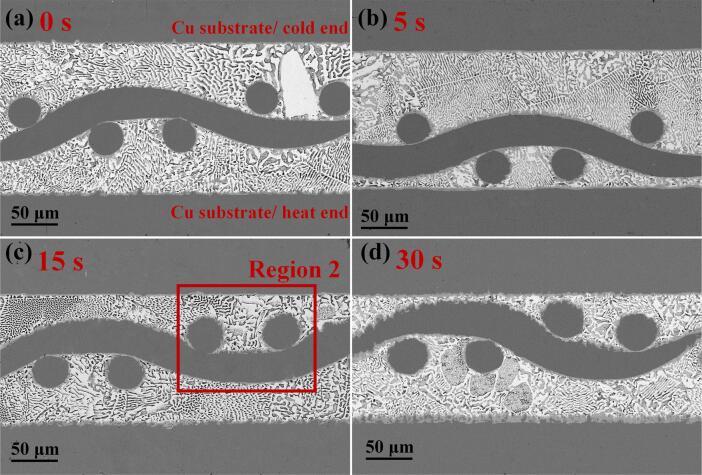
Cu/ Sn58Bi- Ni mesh/ Cu joints with ultrasonic application durations of (a) 0 s, (b) 5 s, (c) 15 s, and (d) 30 s.
